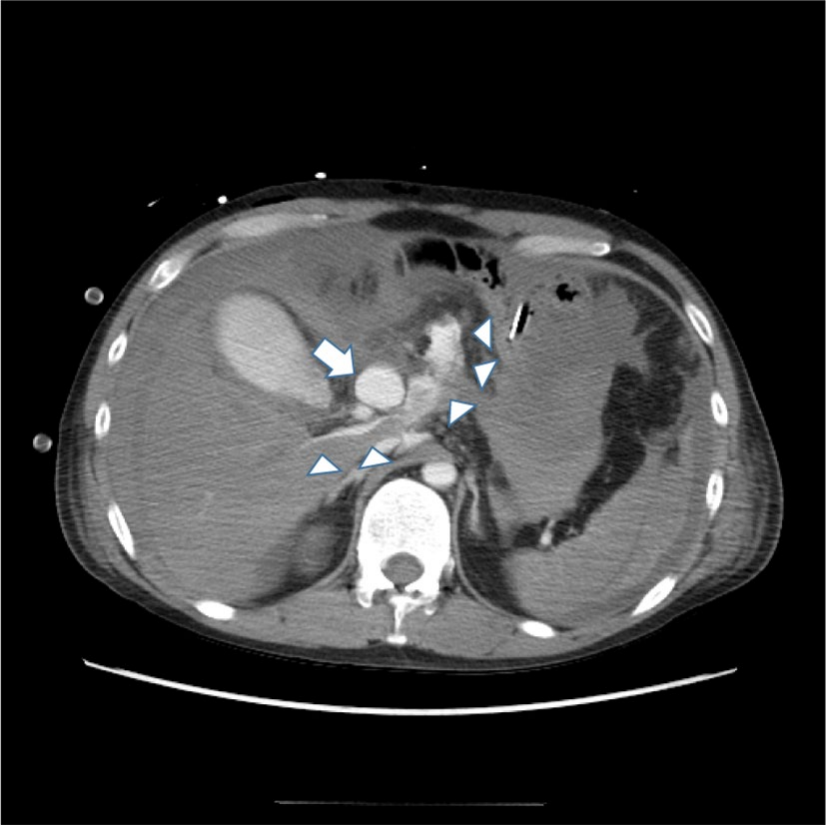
Abdominal computed tomography findings on the fifth day after surgical sigmoid colon resection and colostomy construction. An aneurysm in the common hepatic artery (white arrow) and extravasation (white triangles) are observed
